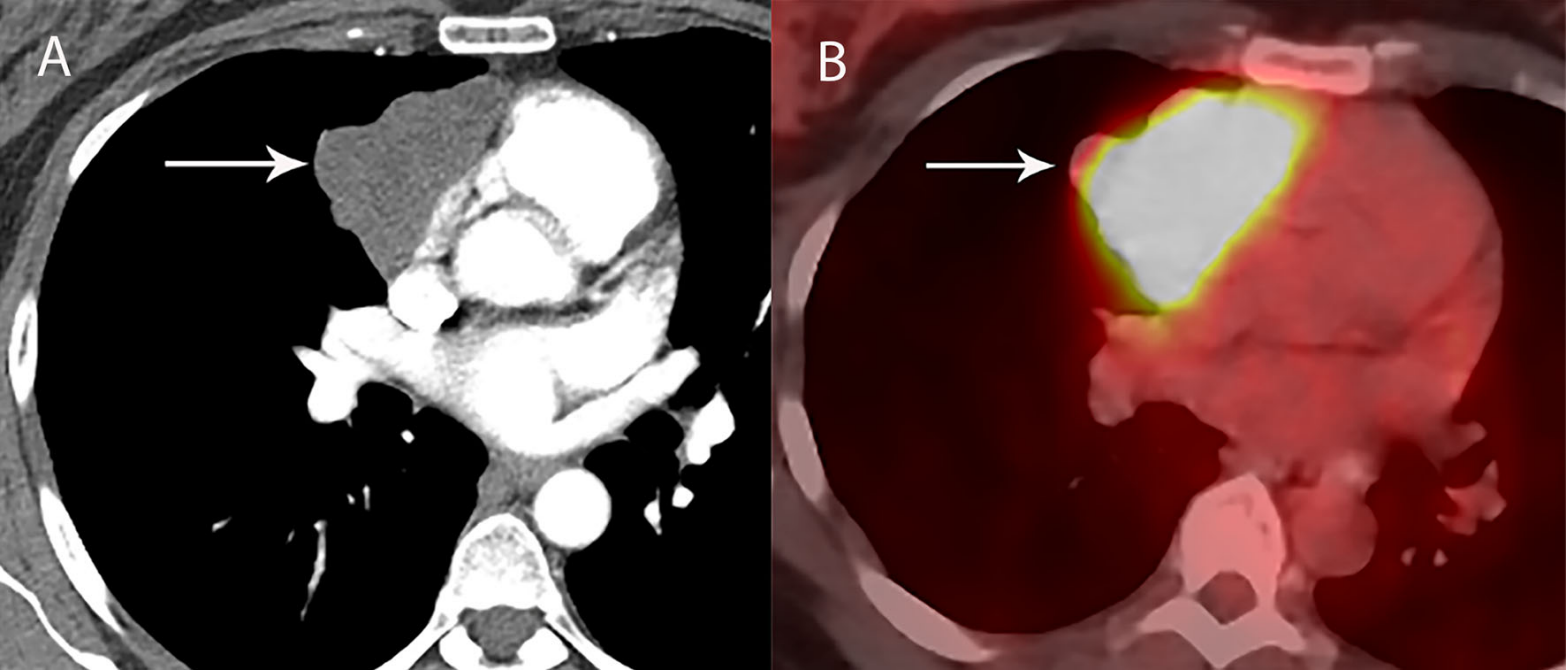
43 year old woman with thymoma. (A) Contrast-enhanced CT shows right prevascular mediastinal mass (arrow). (B) FDG PET/CT shows FDG avid thymoma (arrow) with SUV of 16. Presence of intense FDG uptake suggests more aggressive type thymoma or thymic carcinoma.
